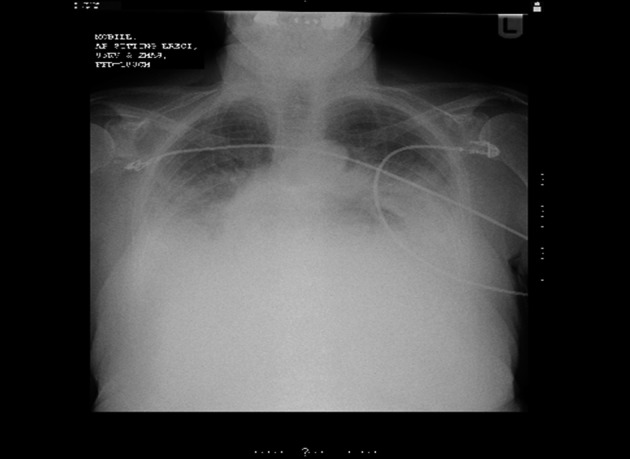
Chest X-ray taken on presentation to the Emergency Department. There is bilateral basal consolidation but no obvious hernia or pneumoperitoneum.
